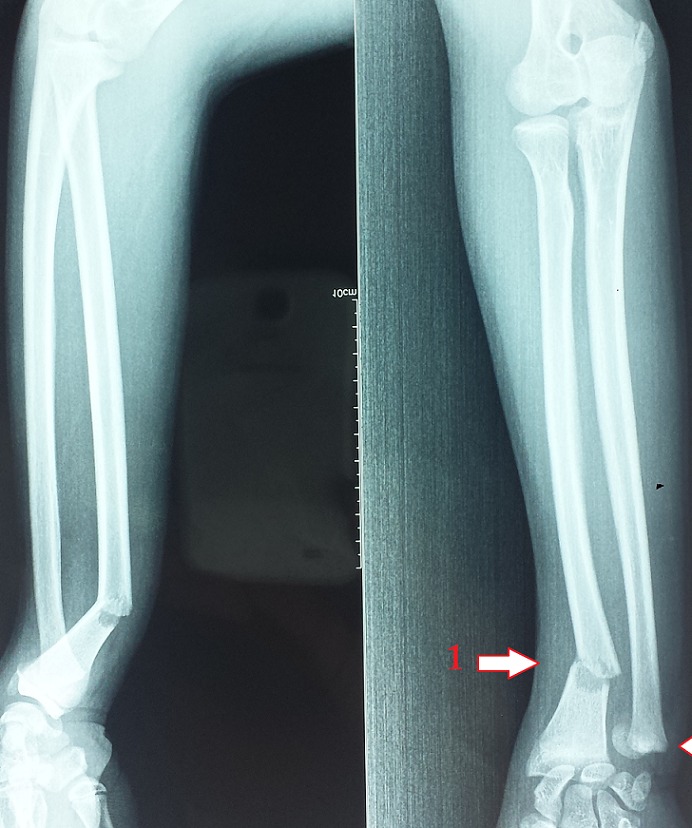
Radiographie de l'avant bras de face et de profil montrant une fracture de la diaphyse radiale avec une angulation antérieure (flèche 1), associée à un décollement épiphysaire stade II de Salter et Harris de l'extrémité distale de l'ulna (flèche 2) soit une fracture équivalente à la fracture de Galeazzi
